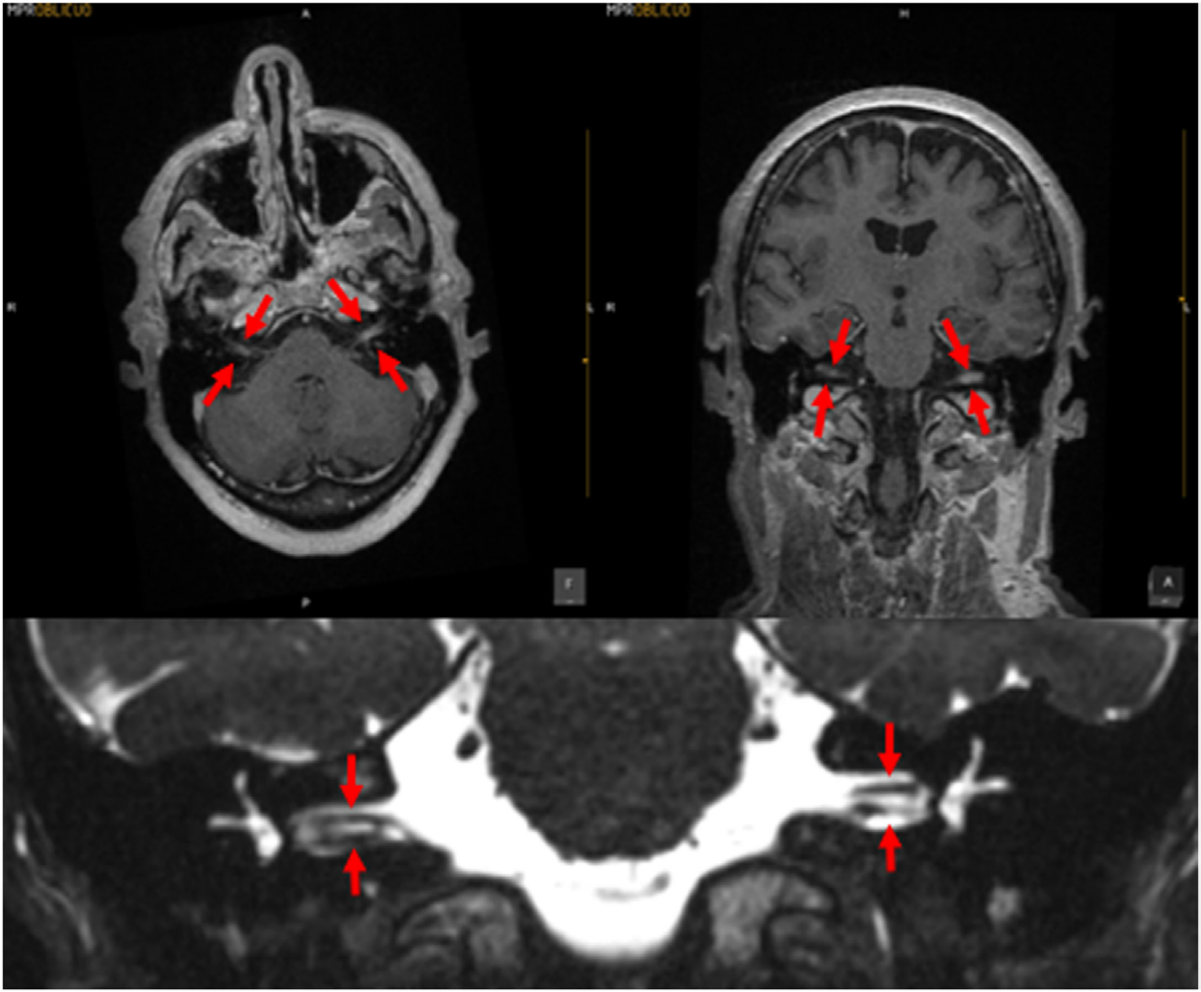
MRI of a patient with meningitis. The upper left panel is a post-contrast T1 axial image; the upper right panel is a post-contrast coronal image; the arrows indicate enhancement of the vestibulo-cochlear nerves. The lower panel displays a coronal CISS sequence image; the structures indicated by the arrows demonstrate that the vestibulocochlear nerves are of relatively normal caliber, with no evidence of vestibular schwannoma. Images courtesy of Dr. Manuel Perez Akly.
